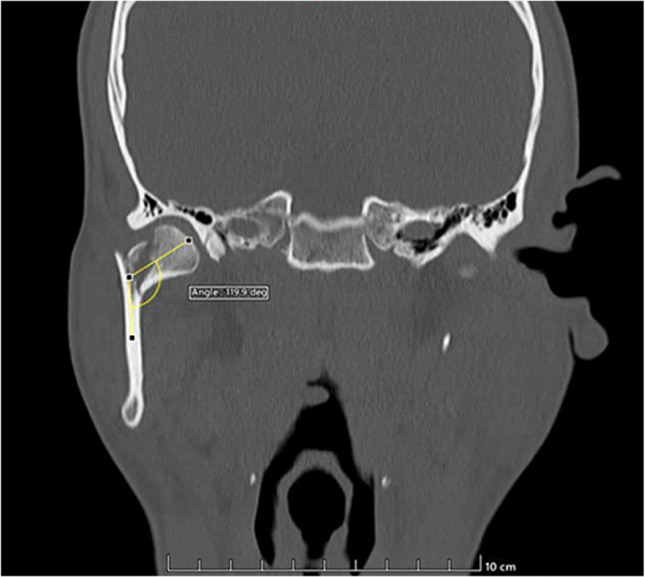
Methodology: Quantification of the Degree of Displacement of Condylar Fracture Bone Stumps. In Facial Computed Tomography, coronal slices containing the most central portions of the posterior margin of the mandibular ramus and the fractured condylar fragment are selected. Thus, two straight lines are projected (yellow color) as virtual extensions of the fractured bone stumps for quantification of the obtuse angle between them using the “Angle tool”, ImageJ software version 18 (National Institutes of Health, Bethesda, Maryland, USA)
